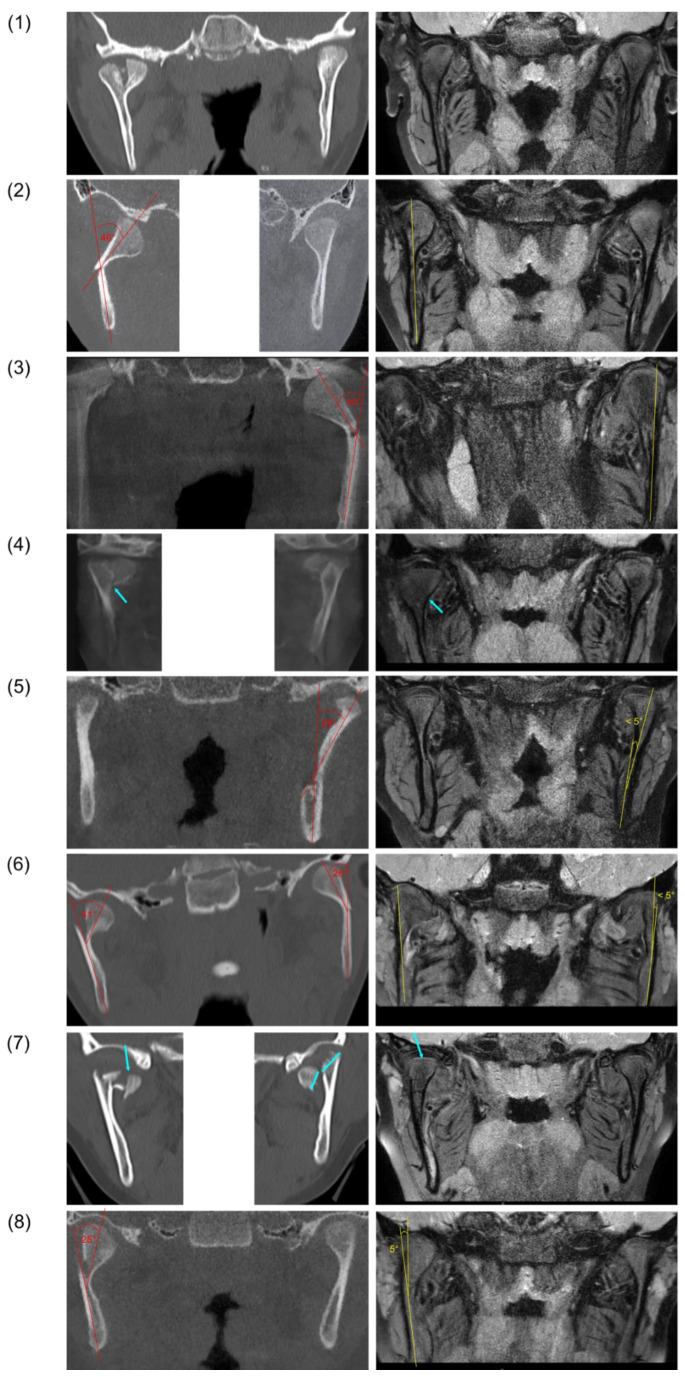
Pretreatment CBCT/CT images (left) and follow-up magnetic resonance images (right) after fractures of the mandibular condyle and FOT in eight patients (rows 1–8). Angulation between fracture fragments is indicated in red (pretreatment) and yellow (follow-up). Fracture lines and bony scar lesions are marked by blue arrows.
